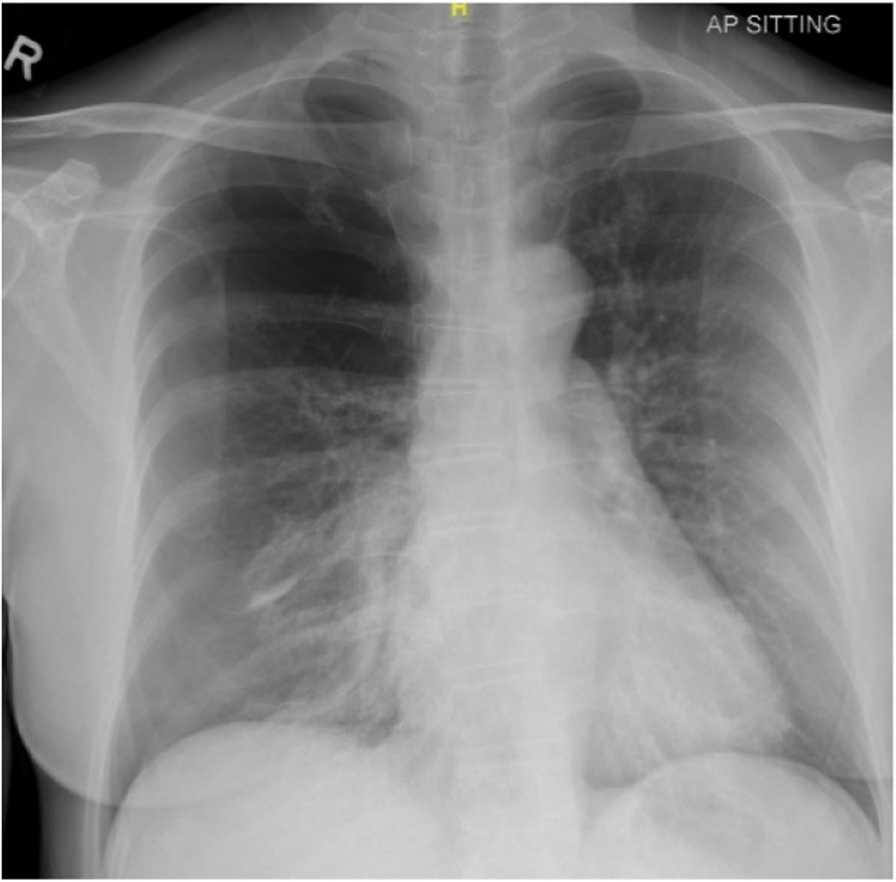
Chest X-ray showing right-sided pneumothorax with apicopleural distance of 7.8cm and left apical pneumothorax with apicopleural distance of 1.7cm. There is no tracheal deviation or mediastinum shift
